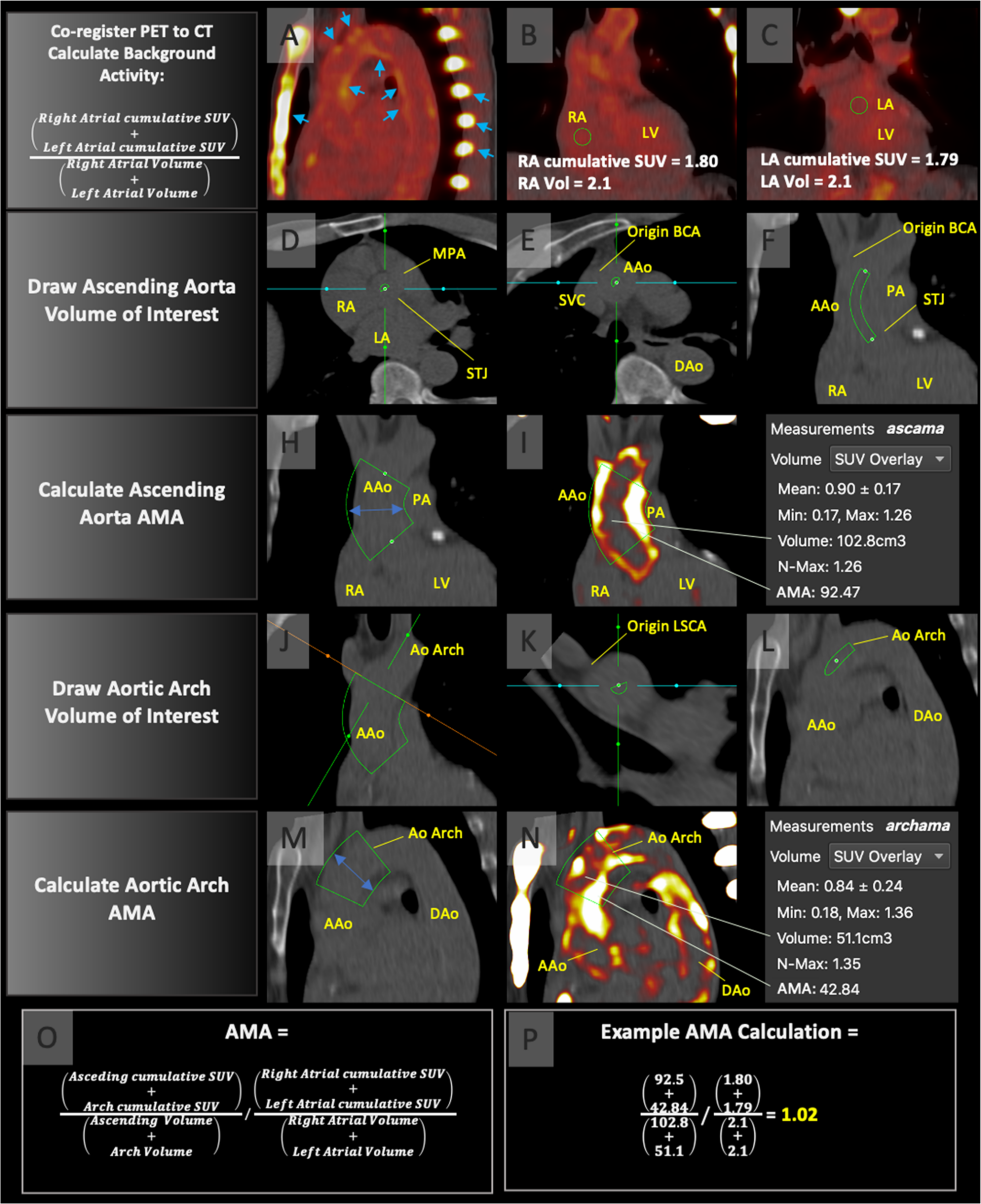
Step-by-step outline of measuring aortic microcalcification activity. (A) Co-register register 18F-sodium fluoride overlay to computed tomography image in three orthogonal planes using landmarks of the sternum, spine, and aortic wall (blue arrows). (B + C) Place a 2 cm^3^ region of interest in the centre of the right (B) and left (C) atrium. The background activity is the cumulative SUV per cm^3^ from the volumes of interest in the left and right atrium. (D, F) With the ^18^F-sodium fluoride overlay turned off, a centreline function is used to draw the ascending aortic volume of interest in multiplanar reconstruction images. Perpendicular to the aorta, the volume of interest starts at the sinotubular junction (D) and finishes at the slice just proximal to the origin of the brachiocephalic artery (E). The width of the volume of interest is increased to the maximum ascending aortic diameter + 4 mm (F + H). The ^18^F-sodium fluoride overlay is reinstated to ensure good coverage (I). The ascending aortic AMA, and volume are calculated (I). The aortic arch volume of interest is drawn with the same method as the ascending aorta, starting with the slice immediately distal to the ascending aortic volume of interest (J), and finishing with the slice after the origin of the left subclavian artery (K). The width of the aortic arch volume of interest is increased to the maximal arch dimeter + 4 mm (M + N). The ^18^F-sodium fluoride overlay is reinstated to check good coverage and calculate the aortic arch AMA and volume (N). (O) Provides the formula for calculating overall AMA, whilst (P) uses the values in the current case to provide a working example of AMA calculation
